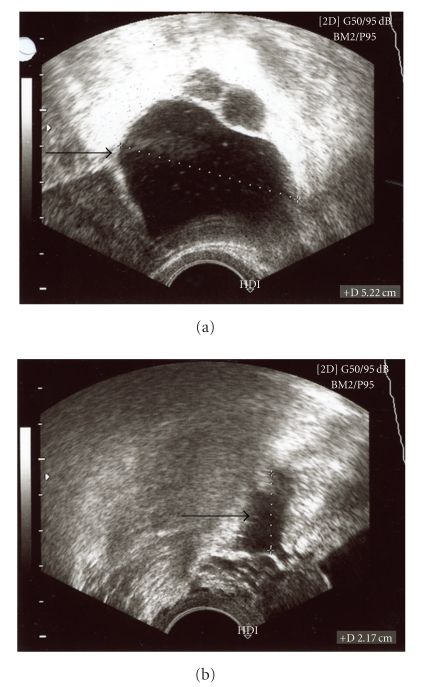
Ultrasound findings. On ultrasound examination a 5 × 6 cm cystic mass lesion in the area of the left fallopian tube could be displayed.
